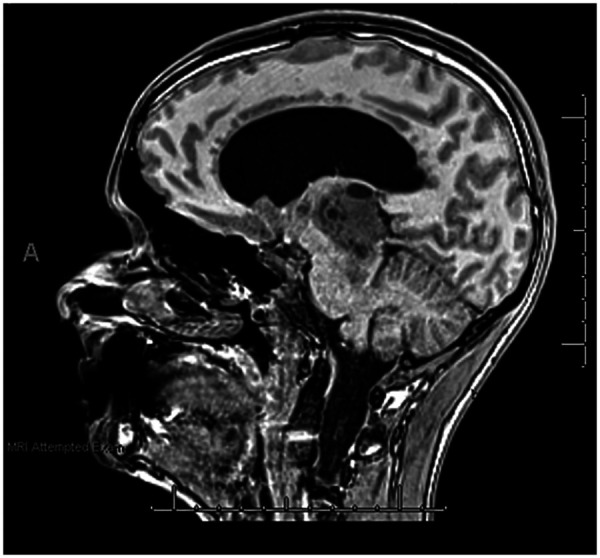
Sagittal T1 Magnetization-Prepared RApid Gradient Echo (MPRAGE) MRI of the patient’s head. The image was taken when the OCD was the most severe (by both youth and parent report).
